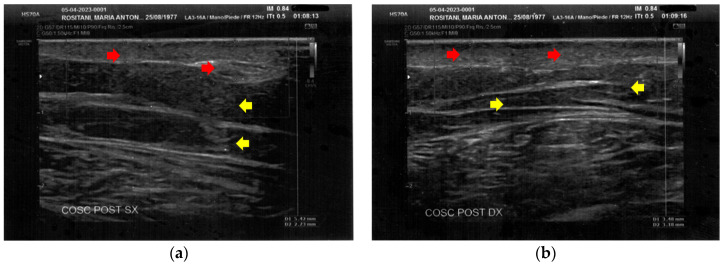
Ultrasonography of the posterior thighs at T0. (a) On the left thigh, cicatricial fibrosis (red arrows) is evident on the superficial layers, and phlogosis extends to the deeper layers (yellow arrows); (b) On the right thigh, cicatricial fibrosis (red arrows) is present as on the left side, and the deeper layers present an inhomogeneous restructuring (yellow arrows).
